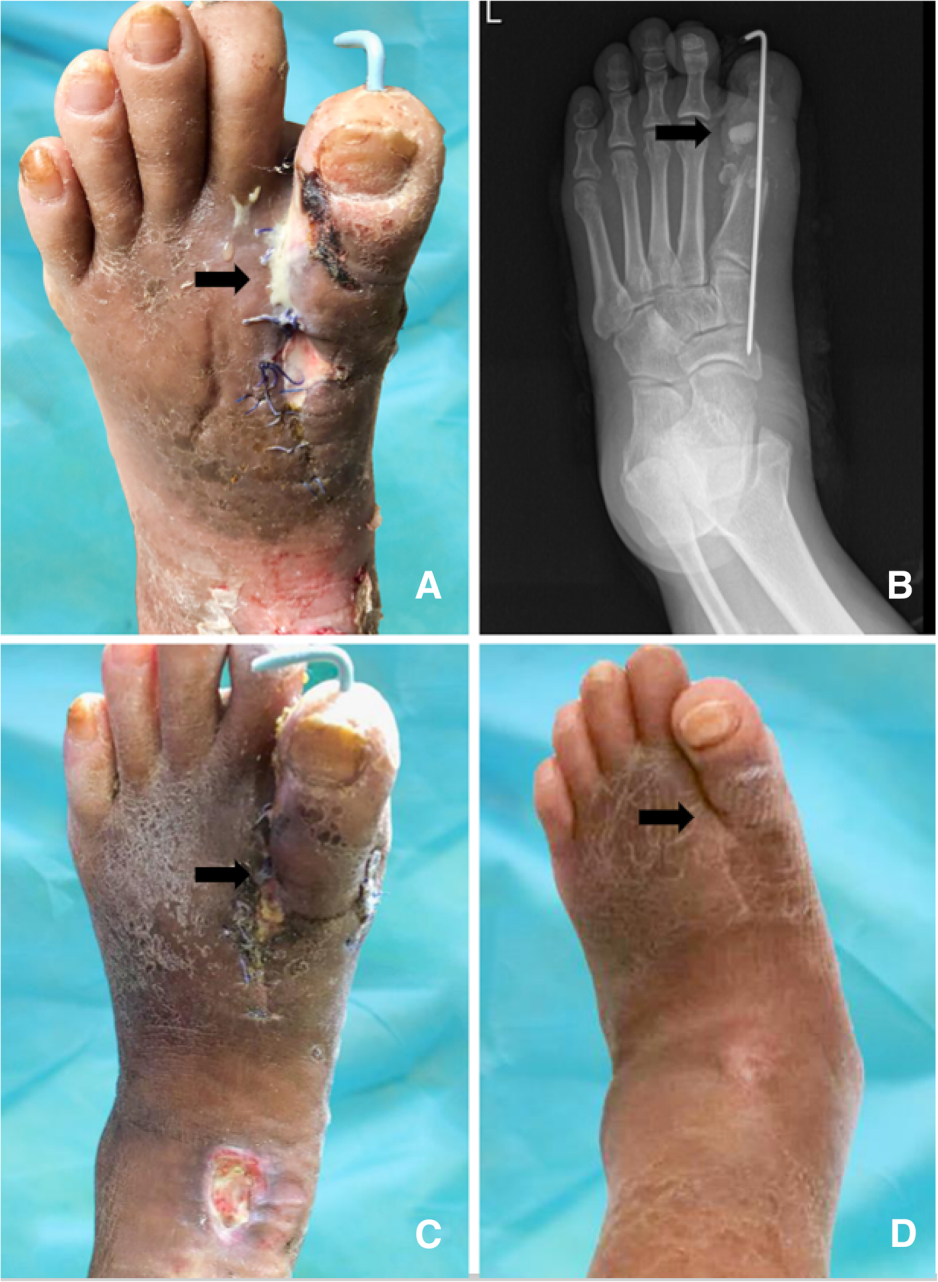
A 62-yaer-old patient with DFO on left first metatarsophalangeal joint. The metatarsophalangeal joint, partial metatarsal and phalange were removed. a The postoperative leakage of calcium sulfate. This sterile leakage was demonstrated as a kind of white, foamy, antibiotics-containing fluid. b X-ray presentation. Vancomycin-impregnated calcium sulfate lump was degrading. c 7 weeks after operation, the skin needed to be moistened. Superficial ulcer in ankle could be managed with dressing. d 1 year after surgical treatment. Although edema was presented, the operative wound healed and the symptoms of infection were disappeared
